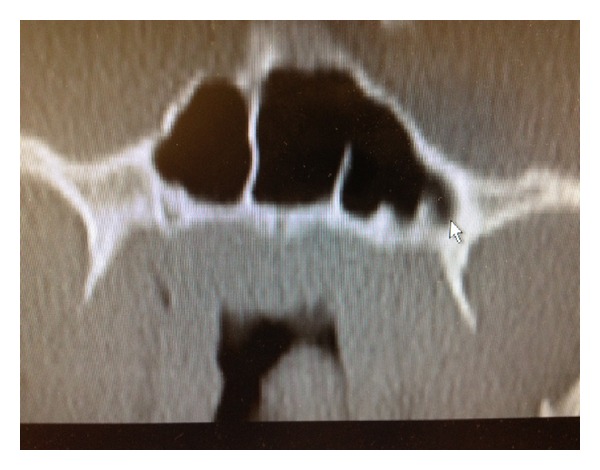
CT coronal image demonstrating a nasopharyngeal mass.
